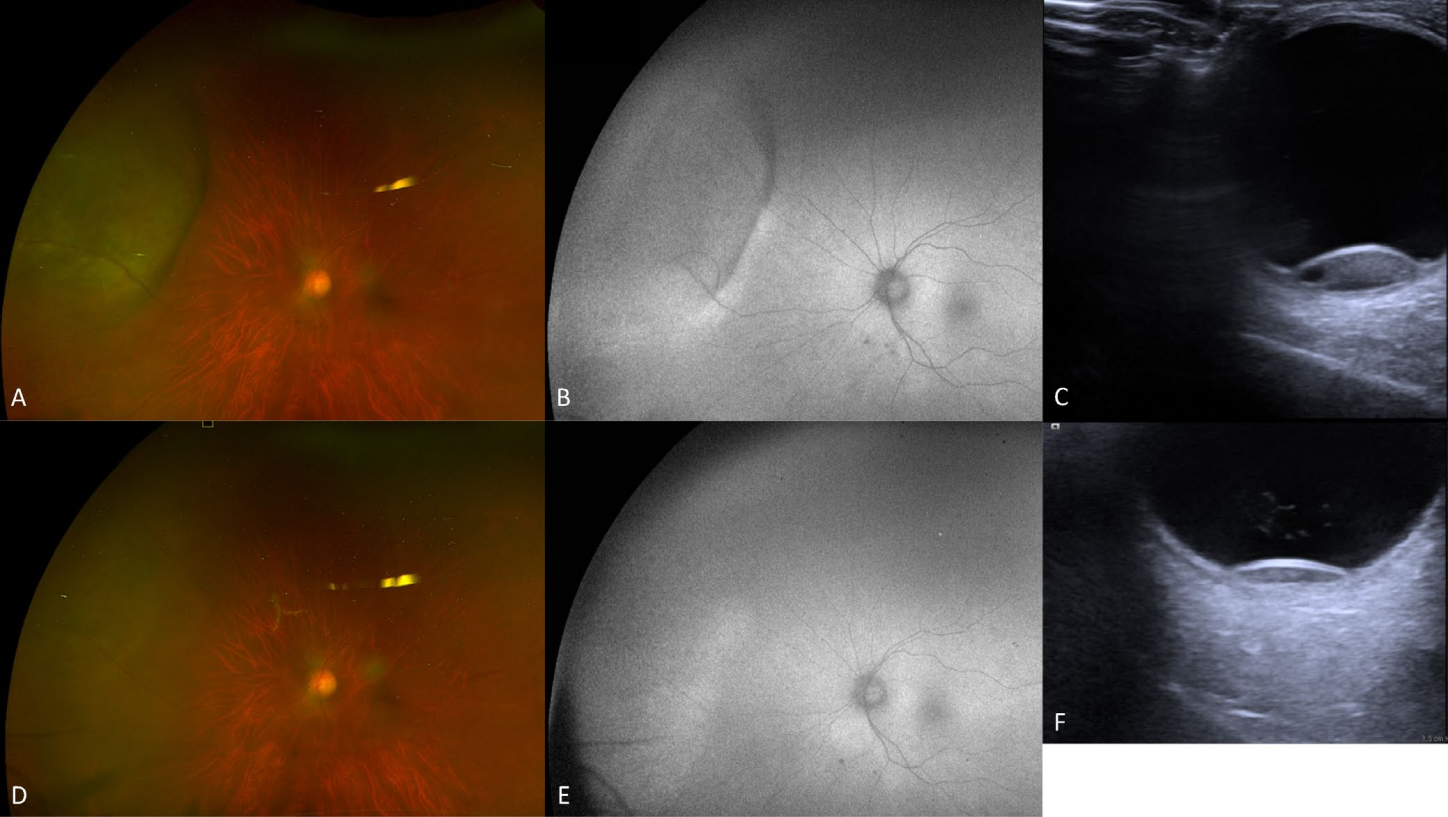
Response of choroidal metastasis to plaque brachytherapy. Pseudocolor image before treatment (A) shows an orange choroidal lesion surrounded by subretinal fluid, as also seen on the autofluorescence image (B). On high resolution ultrasound (C), it has low to medium internal echogenicity, with an echolucent cavity present within the lesion at the nasal border, and 3.4 mm elevation. Three months after the treatment, pseudocolour (D) and autofluorescence images (E) show good tumour control with improvement of the elevation to 1.5 mm (F). Patient consent obtained for the use of images for publication.
